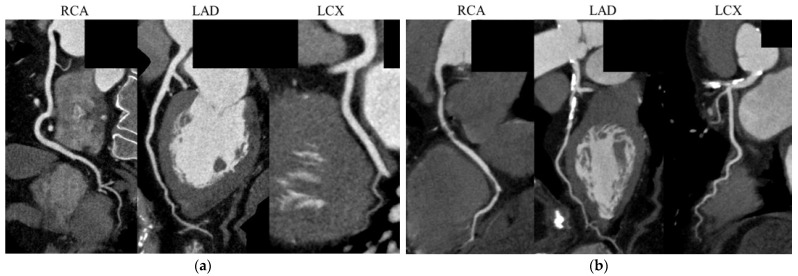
Representative coronary computed tomography angiography images. (a) A 66-year-old man with no noticeable CAD, a FIB-4I level of 0.85, and a Gensini score of 0; and (b) A 78-year-old woman with a FIB-4I level of 3.13 and a Gensini score of 45, showing multiple coronary artery stenoses. CAD, coronary artery disease; LAD, left anterior descending artery; LCX, left circumflex artery; RCA, right coronary artery.
